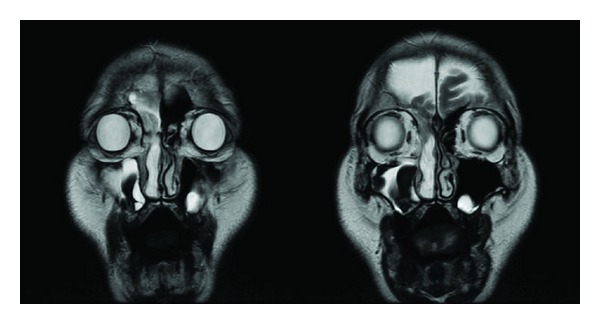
MRI showed that the tumor had high signal intensity surrounded by an isointense area on the T2-weighted image. No connection was observed with the meninges or cerebral tissue.
